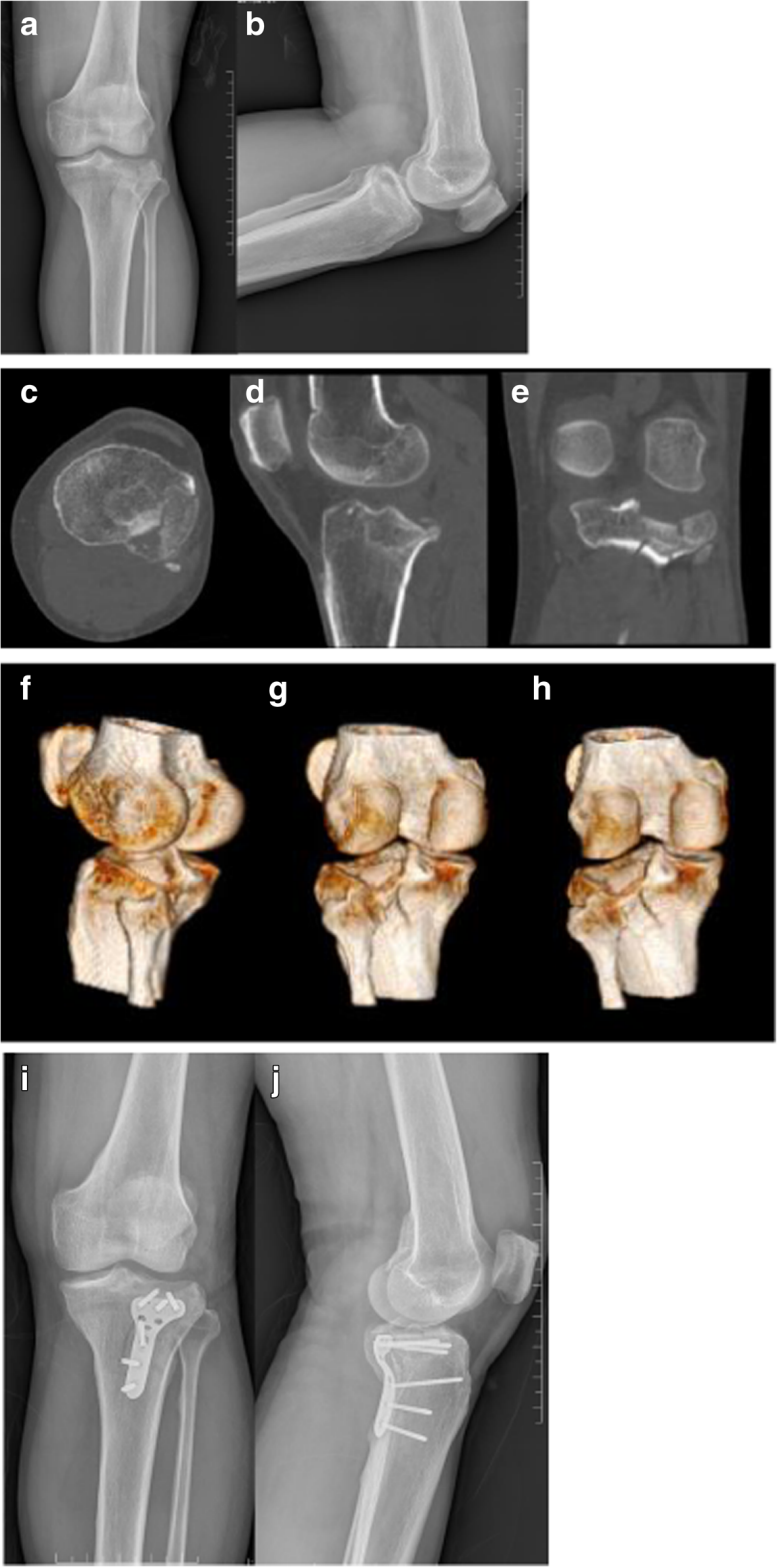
One male patient with posterolateral tibial plateau fracture, 43y, caused by car-accident. a, b were preoperational anteroposterior and lateral radiographs of one posteroleteral tibial plateau fracture. c-h showed more fracture details by 3-D reconstruction of CT scan. i, j were anteroposterior and lateral radiographs of the patient acquired after open reduction and internal fixation with the plate
